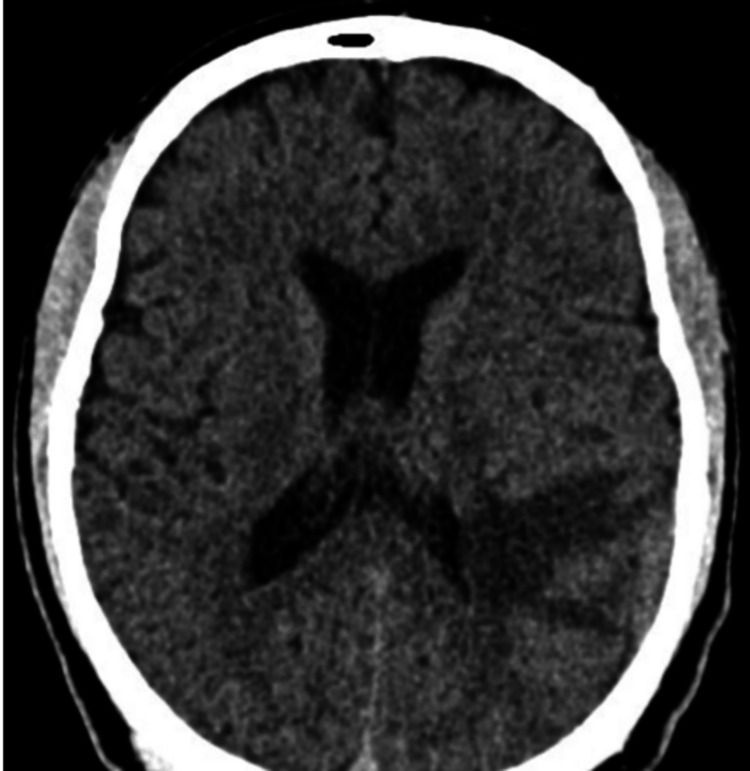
Magnetic resonance imaging Vasogenic edema of the left temporal lobe and inferior parietal lobe.
